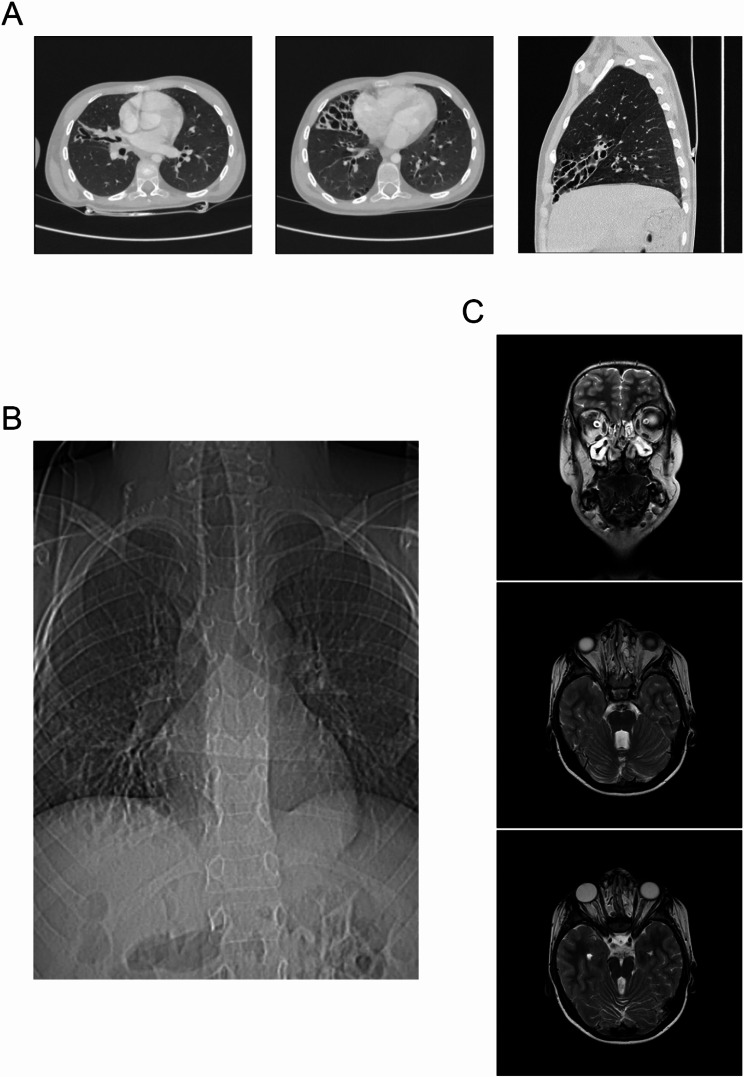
(A) Chest computed tomography scan showing cystic malformation of the middle lobe of the right lung, hiatal hernia with gastric prolapse, subpleural cysts, and bronchiectasis. (B) Esophageal and gastric fluoroscopy with barium contrast demonstrating a sliding hiatal hernia with prolapse of the gastric mucosa. (C) Brain magnetic resonance imaging indicating anomalies consistent with Joubert syndrome, cleft palate, and edema with polyposis of the paranasal sinuses
